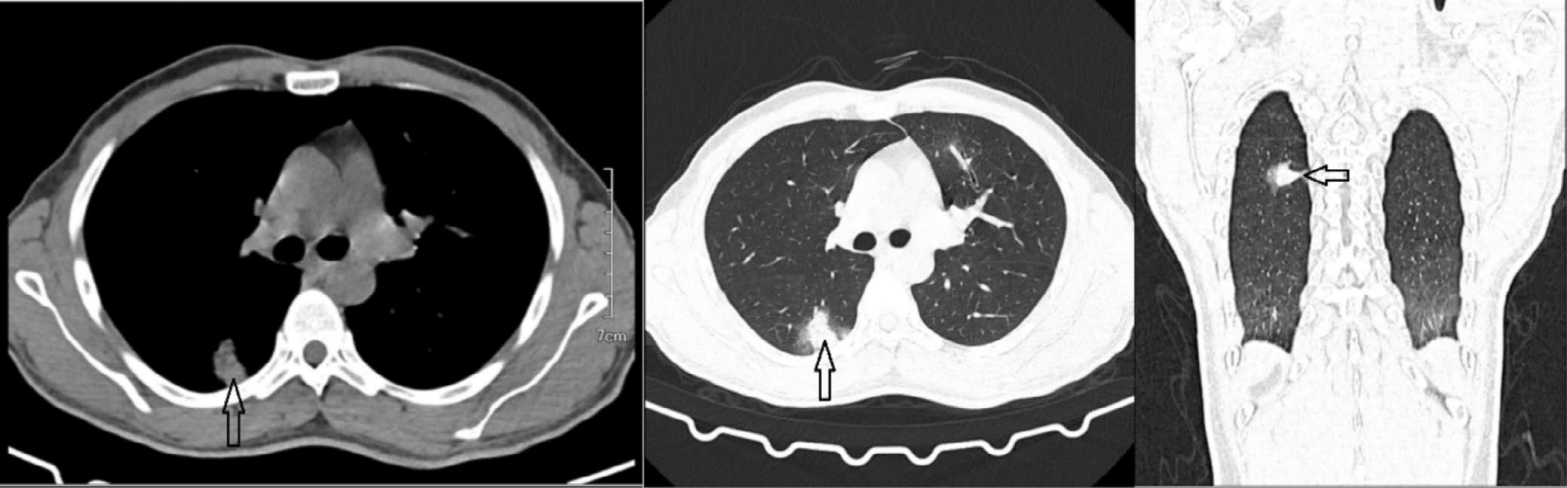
The chest CT scan revealed an 8‐mm nodule in the RLL (highlighted by the arrows).
